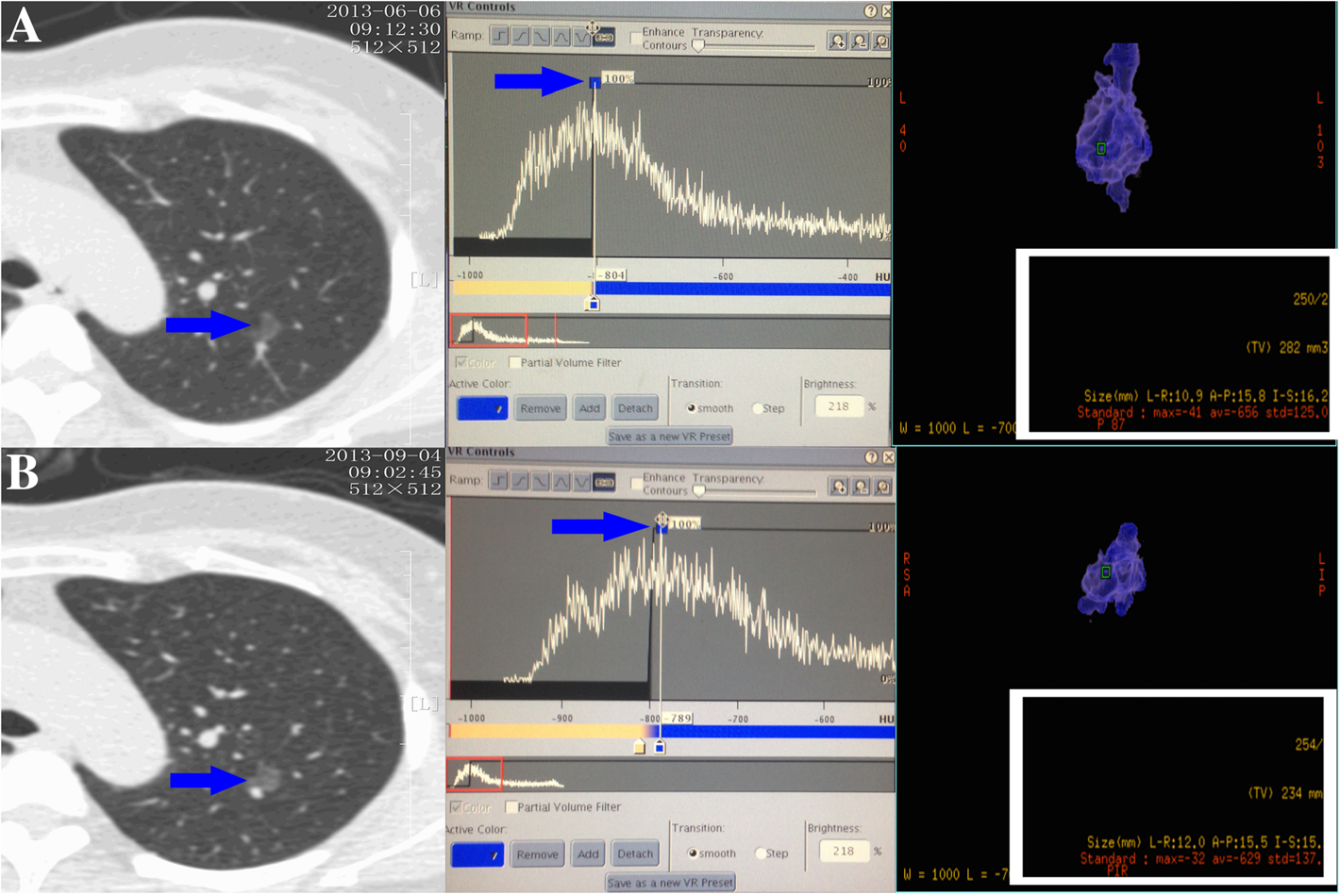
Example of a nodule visualized using computed tomography (CT), CT number histogram and three-dimensional model. a A ground-glass nodule (GGN) located in the upper lobe of left lung (*blue arrow*). The peak (*blue arrow*) CT number in the histogram was −804 HU and the maximum CT number measured automatically in the three-dimensional reconstructive model was −41 HU (*white box*). b The same GGN redetected after a 3-month follow-up (*blue arrow*). The peak (blue arrow) CT number had increased to −789 HU and the maximum CT number had increased to −32 HU (*white box*)
